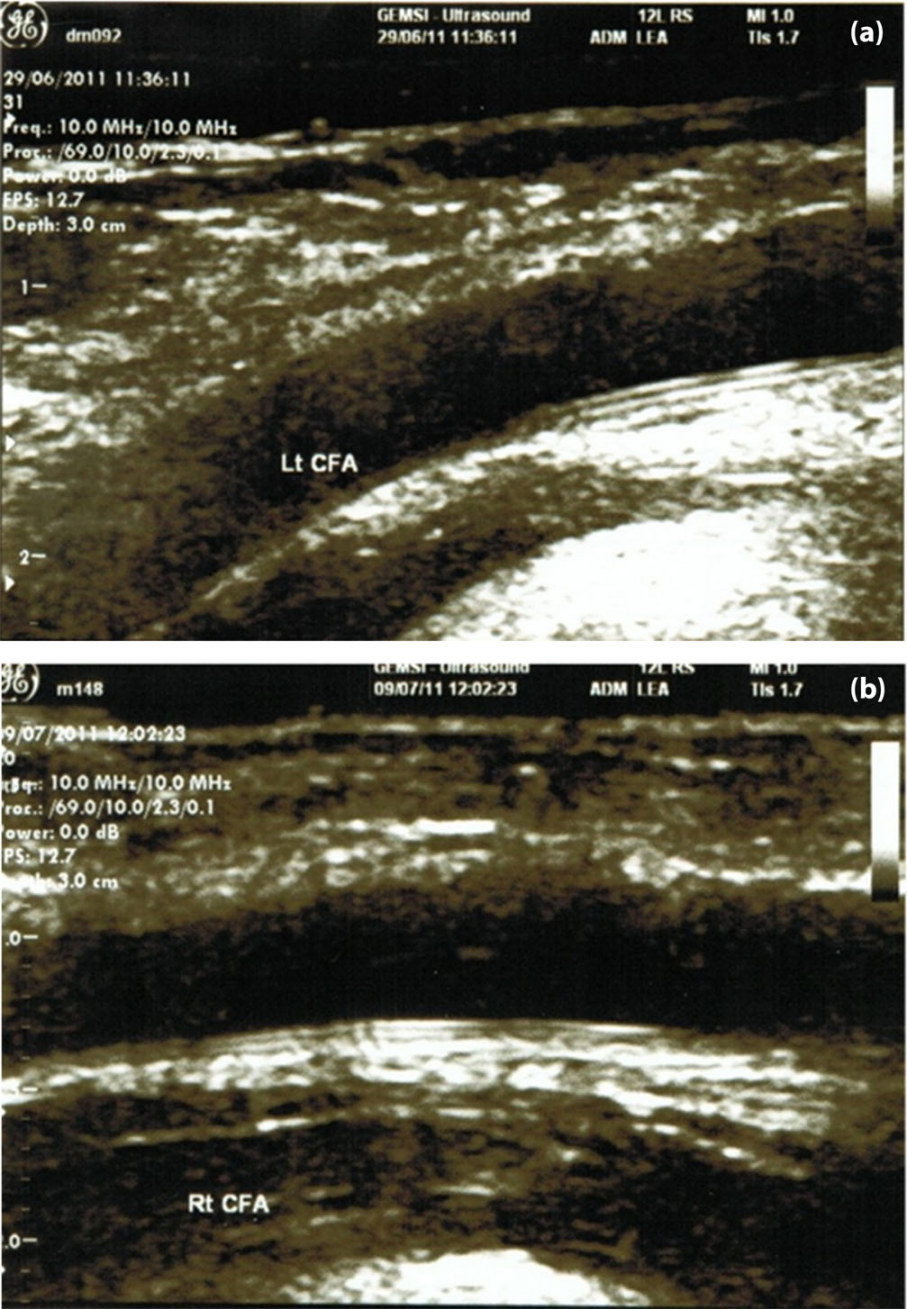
Representative ultrasound images of the triple‐line pattern (TLP) in lower extremity arteries of adolescents with type 1 diabetes. (a) A 21‐year‐old woman with T1D for 13.3 years (BMI 17.0 kg/m^2^, plasma glucose 166 mg/dL, HbA1c 6.5%) demonstrated TLP in the left CFA, but no TLP in the carotid artery. (b) A 18‐year‐old man with type 1 diabetes for 4.5 years (BMI 19.6 kg/m^2^, plasma glucose 77 mg/dL, HbA1c 9.7%) exhibited TLP in both the right common femoral and popliteal arteries, whereas the carotid artery was free of TLP.
